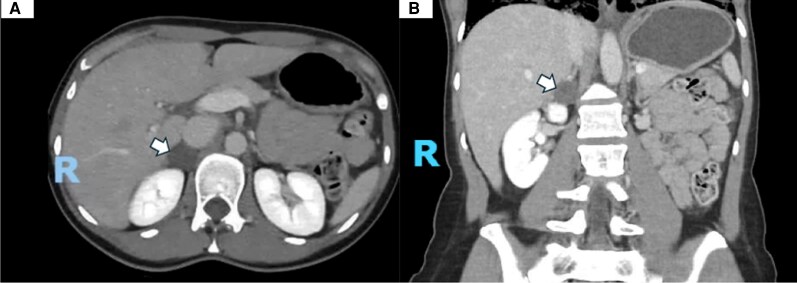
Computed tomography of the abdomen with intravenous contrast. The white arrows demonstrate a 3.0 × 2.2 × 2.6 cm well-circumscribed right adrenal nodular structure of 28 Hounsfield units in the axial view (A) and coronal view (B).
